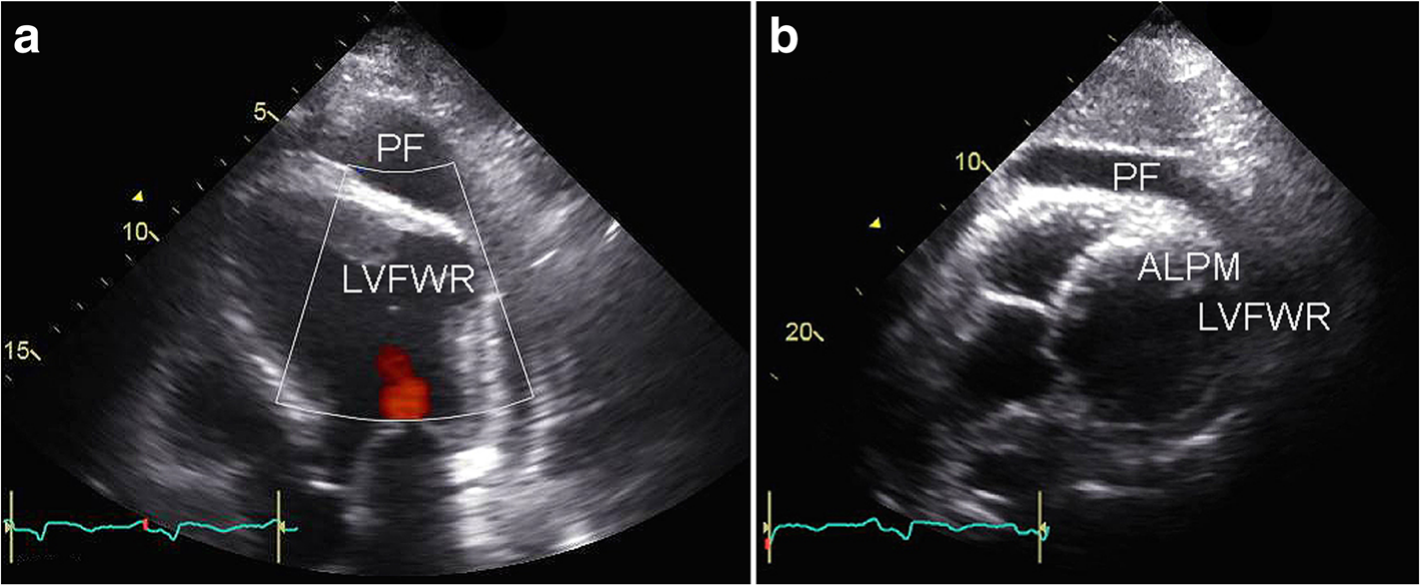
Preoperative transthoracic echocardiographic imaging. Preoperative transthoracic echocardiography showing covered left ventricular free wall rupture (LVFWR) of the lateral wall in the apical four-chamber view (a) and the parasternal short-axis view (b). Note extensive pericardial fluid (PF) and the close proximity of the rupture to the anterolateral papillary muscle (ALPM)
